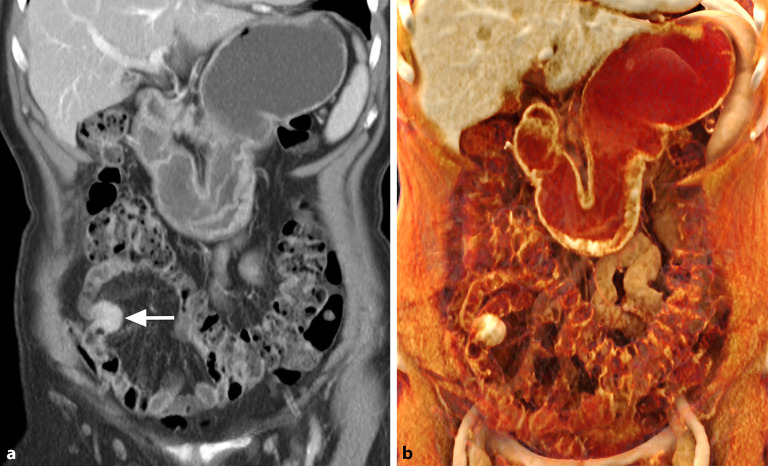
72-jährige Patientin mit unspezifischen abdominalen Symptomen: Die koronale venöse CT(Computertomographie)-Abbildung (a) zeigt einen hypervaskularisierten Tumor des Dünndarms (*Pfeil*) mit charakteristischer Verziehung („Haarnadelzeichen“); b 3-dimensionale Rekonstruktion dieses Tumors
